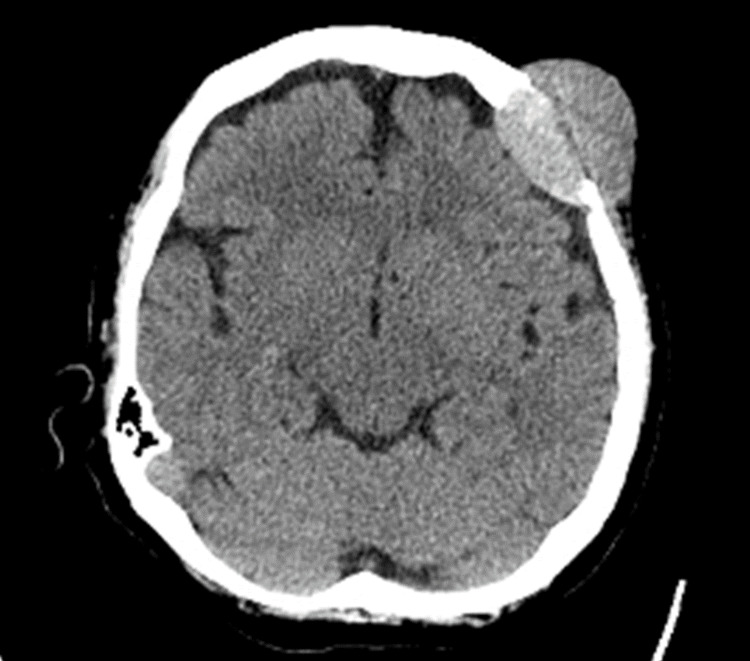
CT head of mass (axial view)
